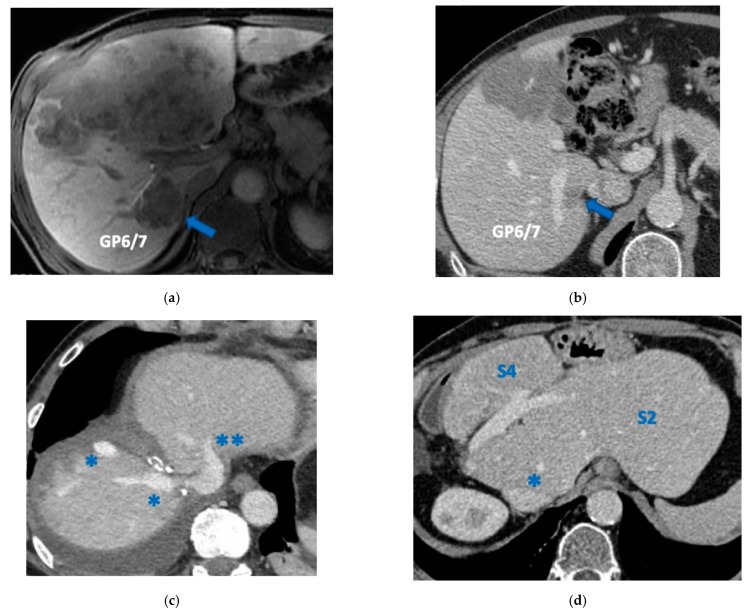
MR and CT images of staged PS-ALPPS performed to treat 16 bilateral CRLM infiltrating the anterior and posterior glissonean pedicle. (a) Hepatobiliary phase of magnetic resonance at diagnosis, blue arrow indicates the CRLM in contact with the posterior glissonean pedicle (GP6/7), posterior bile duct is regular but with a branch inside the CRLM. (b) Partial response after FOLFOXIRI + Cetuximab + Avelumab 8 cycles; blue arrow indicates the CRLM in contact with GP6/7 with massive shrinkage after chemotherapy; we decided to not propose the e-OSH with detachment from GP6/7 preferring the R0 resection; a PS-ALPPS was planned since FLR (=S2/part of S4/Spiegel lobe) volume was 15%; two weeks after the first stage (transaction line preserving part of S4a/S4b and Spiegel lobe and resection of S3, section of MHV and section of right portal vein) FLR increased up to 29% but was judged not enough and an intermediate stage was planned. (c) CT 7 days after intermediate stage (resection of S5/S8), FLR volume increased up to 39% and the third stage was planned to complete the R0 staged PS-ALPPS with simultaneous resection of the primary (left hemicolectomy); * indicates RHV;  indicates LHV. (d) CT after staged PS-ALPPS showing S4, S2 and Spiegel lobe indicated by the single asterisk; 15.5 months after staged PS-ALPPS the patient is disease free; the ALPPS is defined parenchyma sparing since S4 is preserved; * Spiegel lobe. Abbreviations: PS-ALPPS: parenchyma-sparing ALPPS; CRLM: colorectal liver metastases; GP6/7: posterior glissonean pedicle for segments 6 and 7; FLR: future liver remnant; RHV: right hepatic vein; LHV: left hepatic vein; MHV: middle hepatic vein; S: segment.
